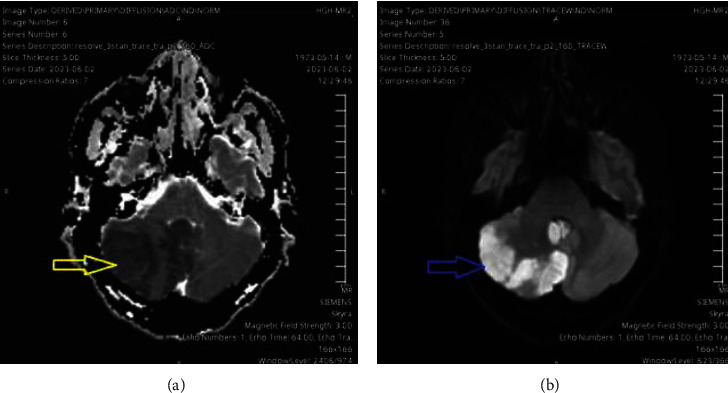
MRI of the head showing the right posterior inferior cerebellar area of diffusion restriction with involvement of the right tonsil: (a) apparent diffusion coefficient (ADC) and (b) diffusion-weighted imaging (DWI).
